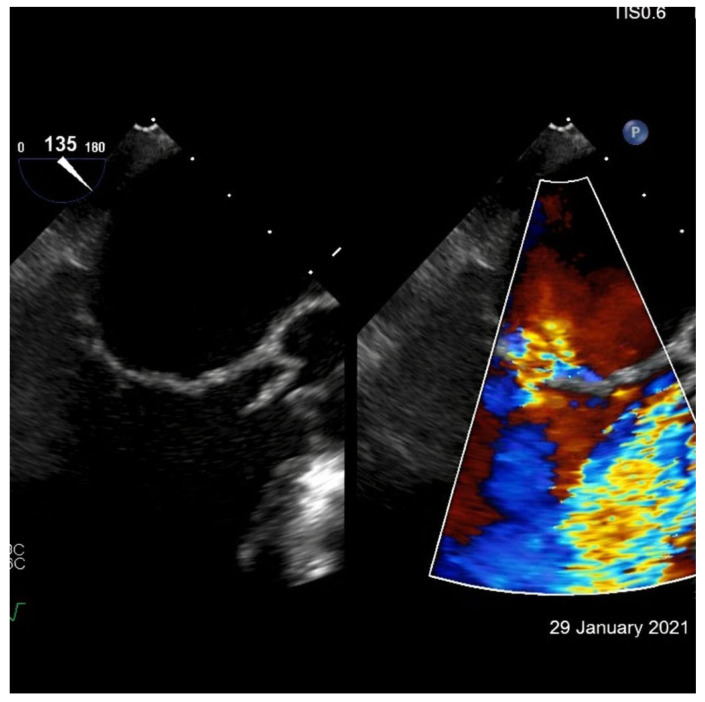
Transesophageal echocardiographic assessment of cardiac hemodynamics. Representative mid-esophageal transesophageal echocardiographic long-axis view with corresponding color Doppler imaging. The grayscale image depicts global ventricular morphology, while color Doppler demonstrates marked intracardiac flow turbulence during diastole, reflecting altered ventricular filling dynamics. These qualitative findings are concordant with impaired left ventricular systolic function, abnormal myocardial deformation, and elevated filling pressures as quantified by left ventricular ejection fraction, global longitudinal strain, and diastolic indices.
